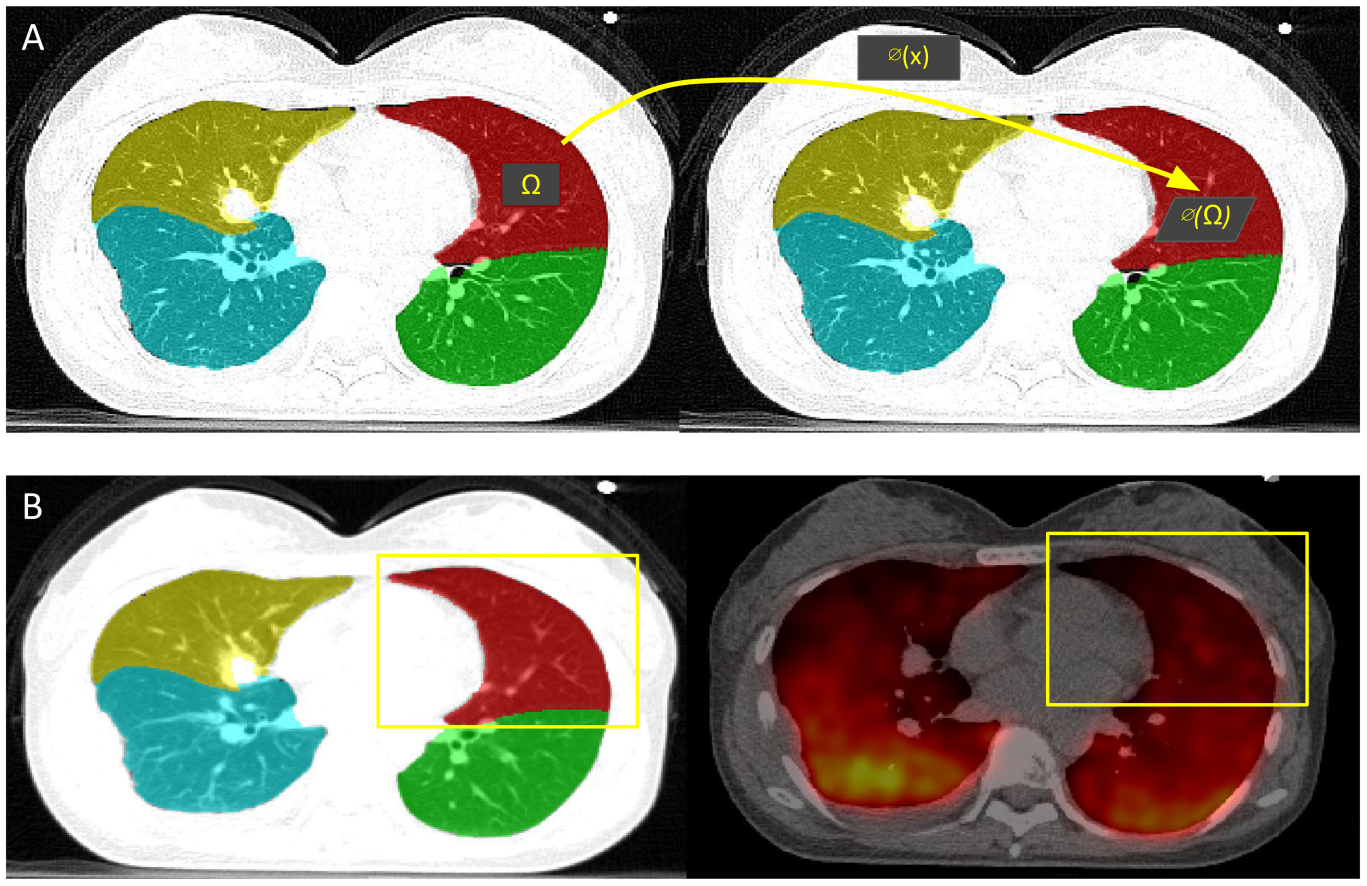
CTVI calculation and validation. (A) CTVI is calculated based on the change in volume between the inhale (right) and exhale (left) segmentations, where ventilation(Ω) = |1 - vol(∅(Ω))/vol(Ω)|. (B) The ground-truth PET-Galligas (right) is acquired by isolating the number of counts per lobe based on the TriSwinUNETR lobe segmentation from the PET-CT scan (left).
